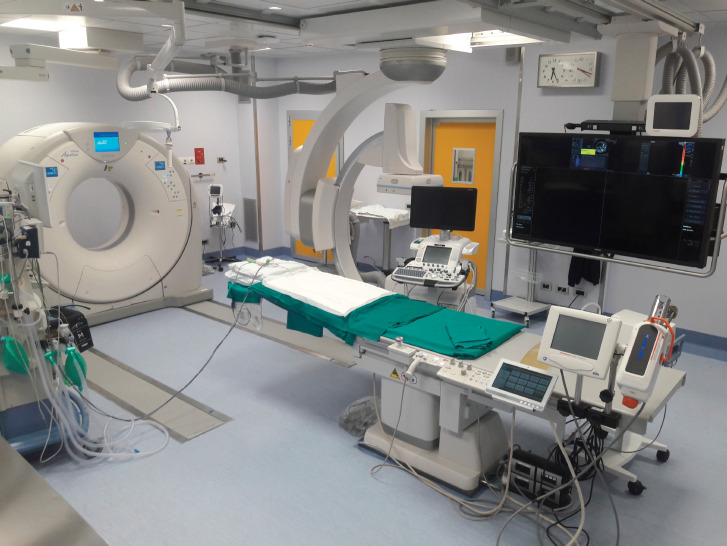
Angio-CT Hybrid Suite with the availability of angiography, CT and US.
